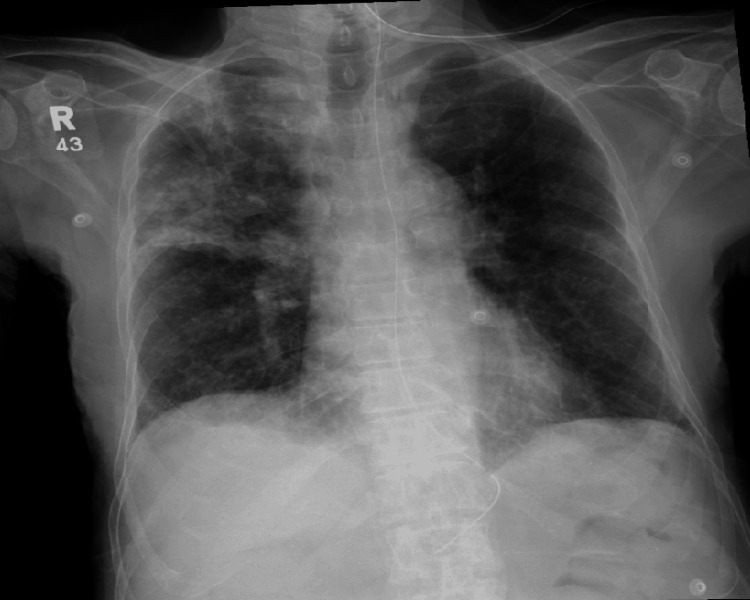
Chest X-ray showing opacity in the right lung
